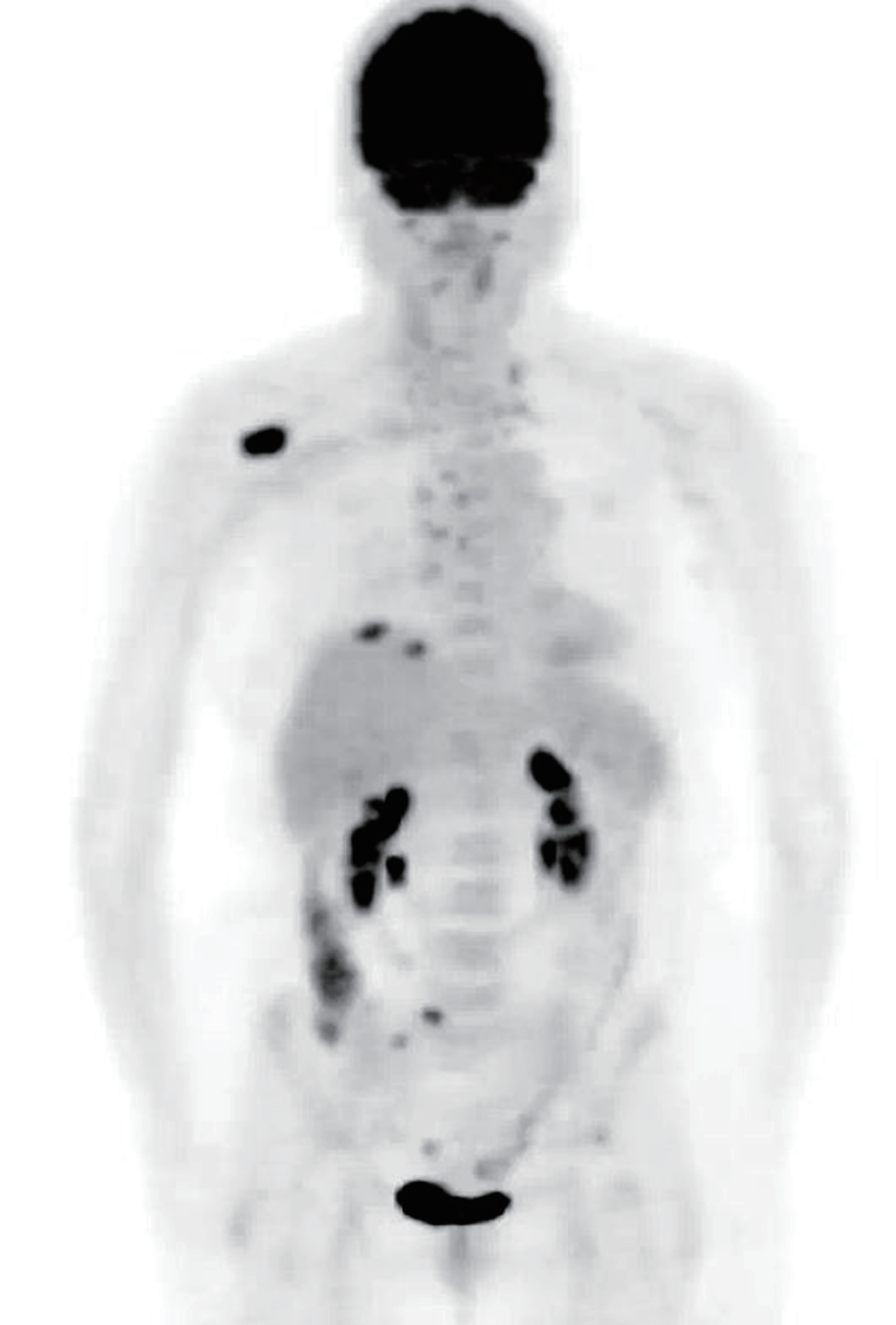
Computed tomography-positron emission tomography performed prior to treatment. Maximum intensity projection image shows mass within right lung base. Adenopathy with abnormal uptake is present throughout the mediastinum and neck. Largest bone metastasis involves right scapula.
